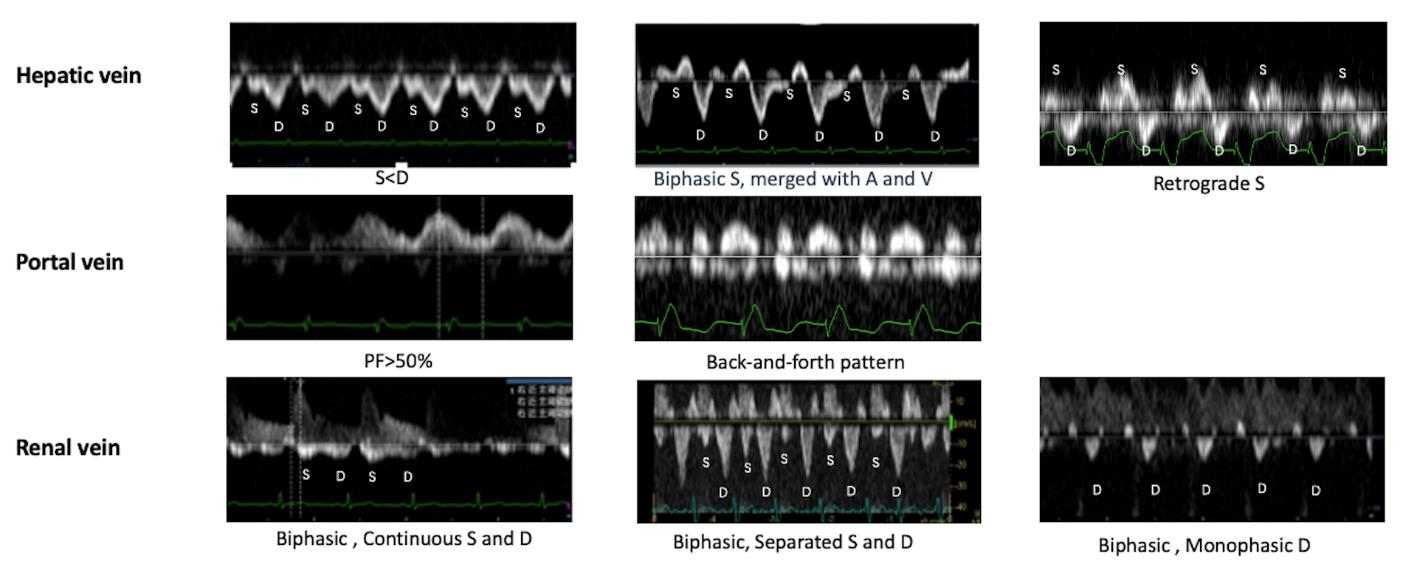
Abnormal systemic VRS in patients with RVD. This figure represents Doppler patterns on hepatic vein, portal vein, renal vein in patients with RVD, and the severity gradually increased from left to right. The changes of the hepatic vein are manifested as:S-wave<D-wave, biphasic S-wave (mergerance with A-wave and V-wave) and retrograde S-wave. The changes of the portal vein are manifested as high portal pulsatility fraction, including PF>50% and back-and-forth pattern. The changes of the renal and intrarenal vein are manifested as “HV-like” flow pattern, including biphasic with continuous S-wave and D-wave, biphasic with separated S-wave and D-wave, biphasic with monophasic D-wave; RVD, right ventricular dysfunction.
